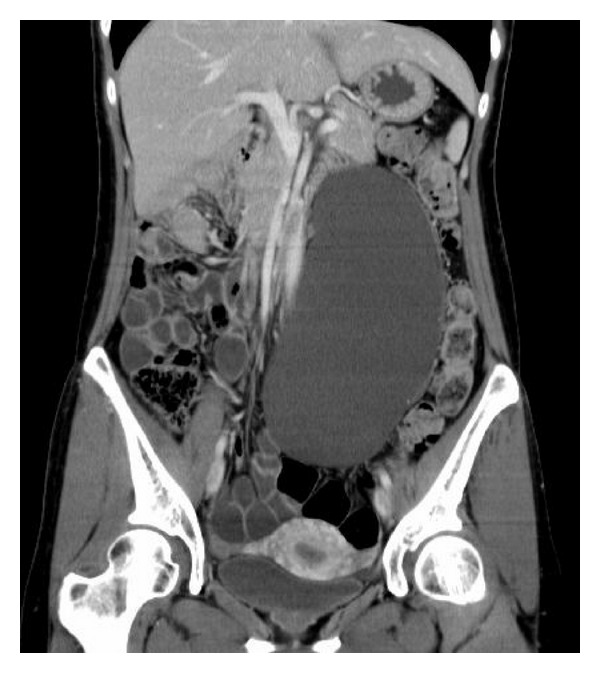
Coronal section of abdominal CT demonstrating a large water density occupying much of the left retroperitoneal space.
